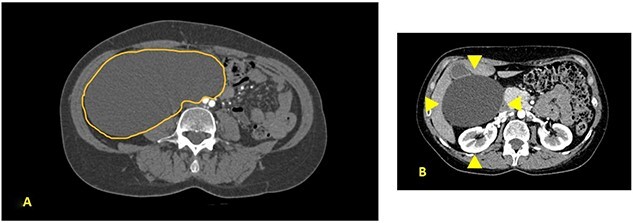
(A) Contrast CT scan; gross oval-shaped mass with clear fluid content (10–15 HU) and regular margins, measuring 15 × 10 cm in maximum diameters; (B) evidence of liver and gallbladder compression (arrowheads).
